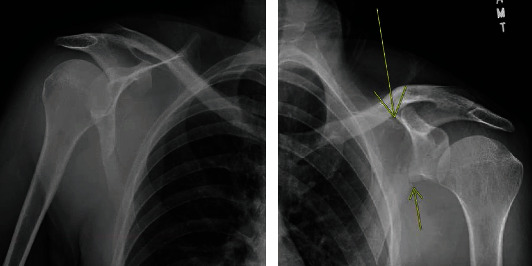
Plain film radiographs of the right shoulder with no obvious right bony abnormalities noted. Left-sided scapular body fracture identified with intact glenohumeral joint.
